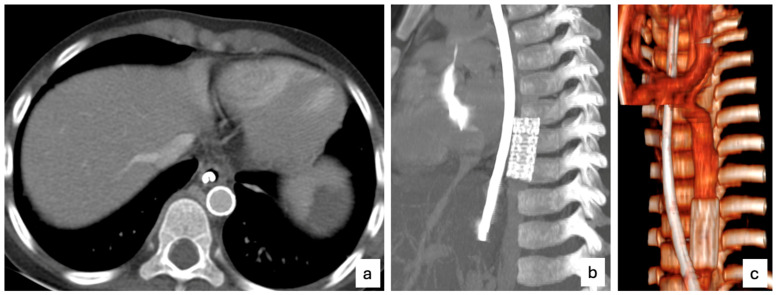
(a) Axial CT scan showing the covered stent correctly positioned in the distal thoracic aorta, with no evidence of extravasation, suggesting complete exclusion of the fistulous tract. The esophagus lies adjacent to the stented segment, without peri-esophageal collections. (b) Sagittal reconstruction demonstrating a well-expanded and properly apposed covered stent, with no signs of endoleak. (c) 3D volume-rendered reconstruction confirming the appropriate alignment and morphology of the aortic stent, with complete exclusion of the involved aortic segment and no parietal irregularities.
